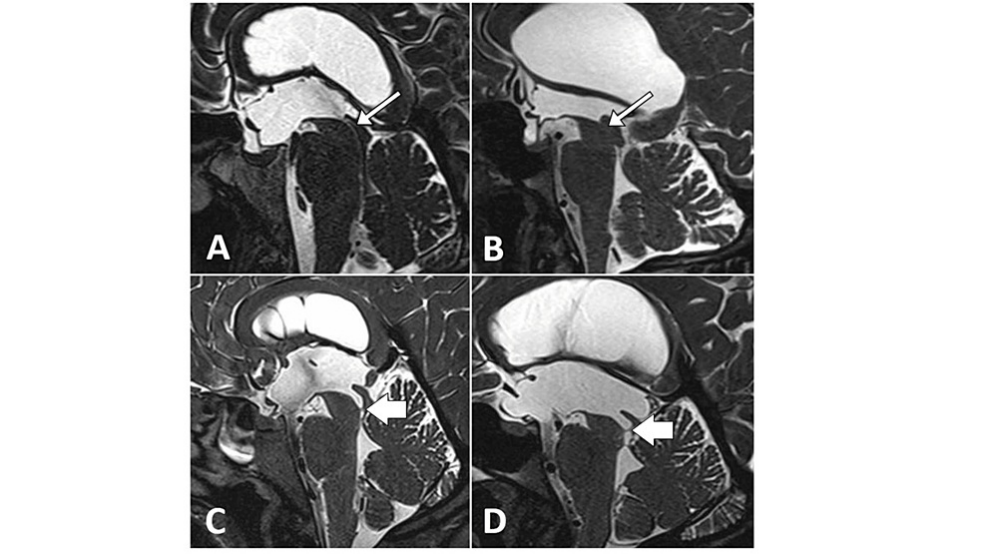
Preoperative CISS sequence MRI sagittal sections in four patients. (A, B) MRI for patient numbers 12 and 15 and (C, D) 17 and 20. The thin arrow points to aqueductal stenosis, and the thick arrow points to the aqueductal web. CISS = constructive interference in steady state
